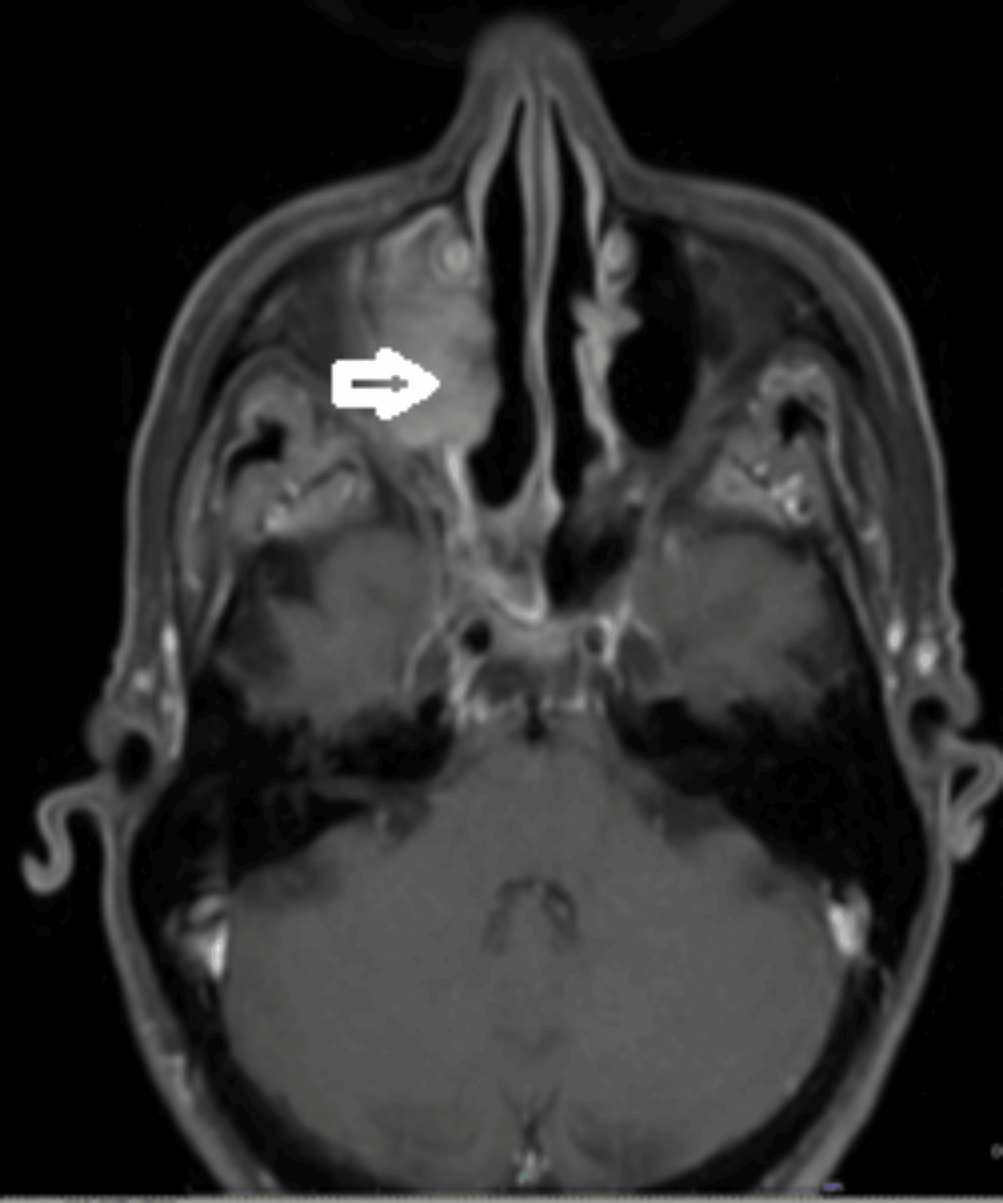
MRI sinus showing tumour extending into the nasal cavity.
